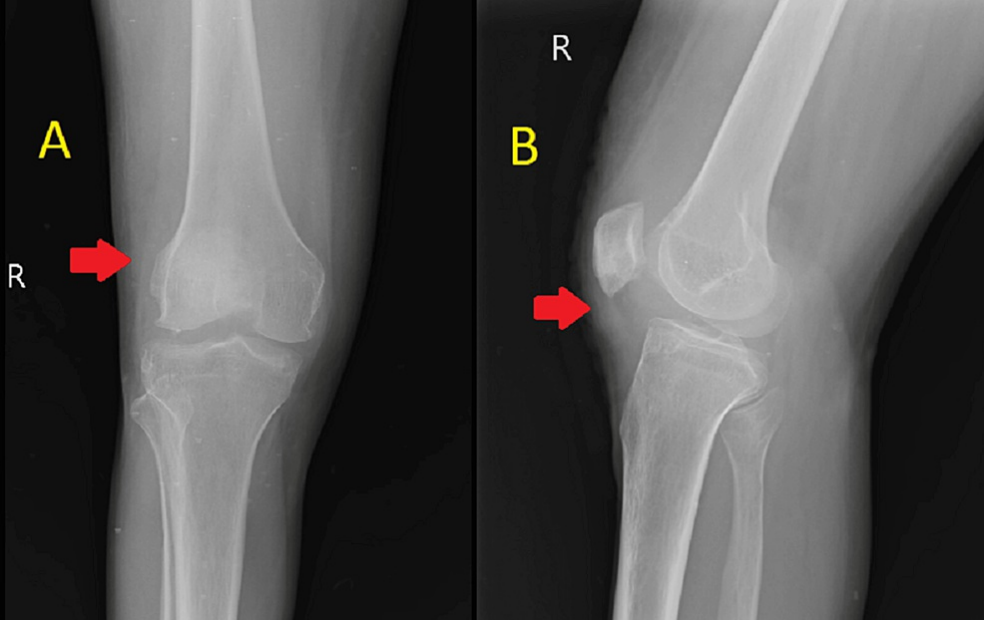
Postoperative radiograph anteroposterior (A) and lateral (B) views of the knee joint Images A and B show the successful excision of multiple loose bodies which were seen in preoperative radiograph at the same site depicted by arrows
